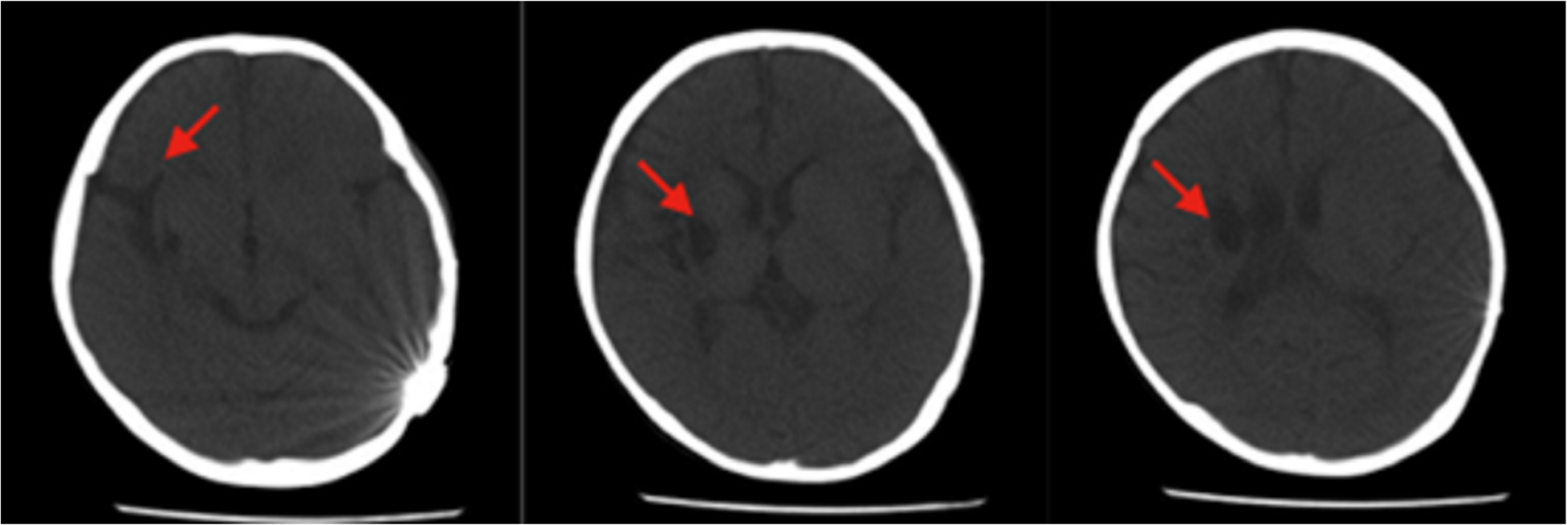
The head CT scan revealed right basal ganglia softening (indicated by an arrow) and right hemisphere cerebral atrophy
